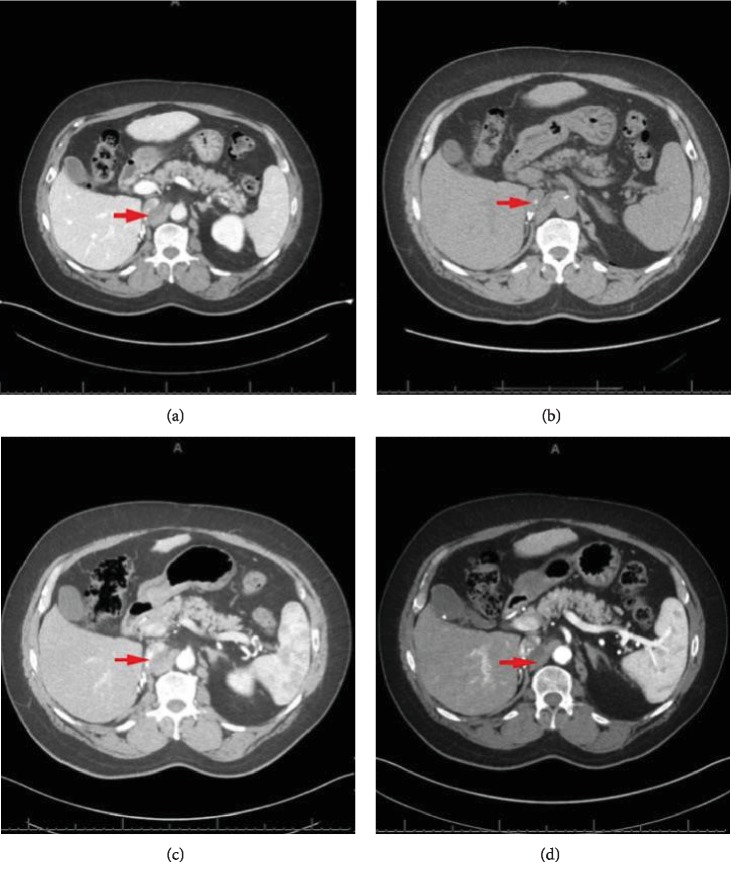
(a) CT abdomen and pelvis w/ contrast; ~1.5 months post discontinuation of nivolumab with the following findings: arrow showing a heterogenous density 3.5 × 1.0 cm lesion in the right retroperitoneal space unchanged from prior imaging (around time of acute pneumonitis occurrence). (b) CT abdomen and pelvis w/ contrast; ~4 months post discontinuation of nivolumab with the following findings: arrow showing a 3 × 1.0 cm soft tissue lesion posterior to inferior vena cava, stable in size. (c) CT abdomen and pelvis w/ contrast; ~9 months post discontinuation of nivolumab with the following findings: arrow showing a 3.1 × 1.3 cm soft tissue lesion posterior to inferior vena cava, stable in size. Of note, supra renal inferior vena cava tumor thrombus was noted measuring 2.8 × 1.5 cm. (d) CT abdomen and pelvis w/ contrast; ~18 months post discontinuation of nivolumab with the following findings: arrow showing a 2.8 × 1.5 cm soft tissue lesion posterior to inferior vena cava, stable in size. The supra renal inferior vena cava tumor thrombus measured at 2.1 × 1.4 cm, improved from prior imaging.
